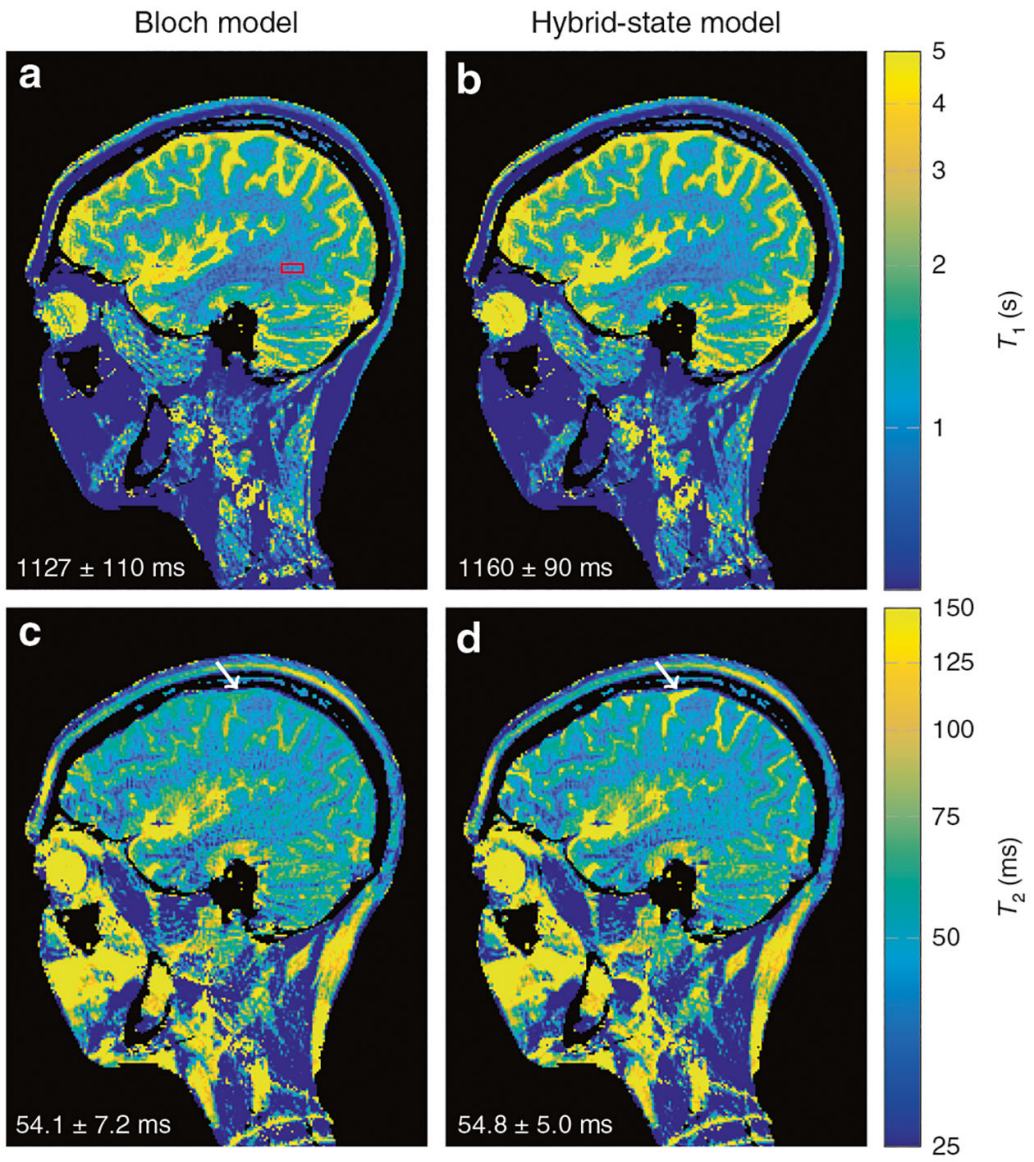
In vivo validation of the hybrid-state model. A single sagittal slice of a 3D magnetic resonance imaging experiment of a human brain is depicted. a, c The data were acquired with an anti-periodic hybrid-state experiment and were fitted with the Bloch model (Eq. (1)), and b, d with the hybrid-state model (Eqs. (5–7)). The parameter maps have a resolution of 1 mm × 1 mm × 2 mm and spatial encoding was performed with a 3D stack-of-stars *k*-space trajectory^62^. The red box indicates a region of interest used for extracting the *T*_1_ and *T*_2_ values denoted in the corner of the images. The arrows indicate a region with very long relaxation times in which slight deviations between the models can be observed. Note the logarithmic scale of the color coding
